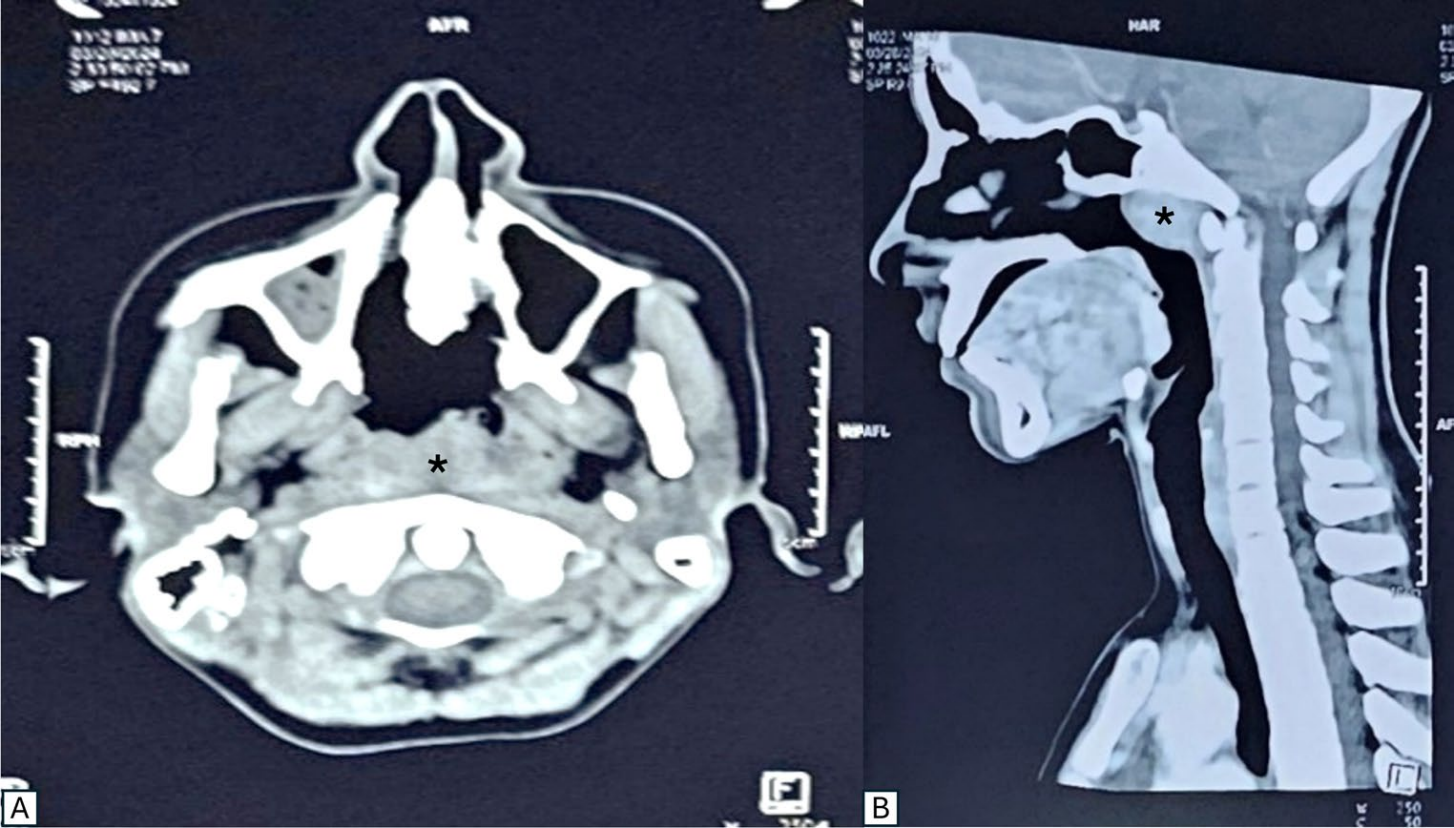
Computed tomography image (A) coronal section and (B) saggital section showing a well-defined, lobulated, heterogeneously enhancing lesion (asterisk) arising from the posterosuperior nasopharyngeal wall
